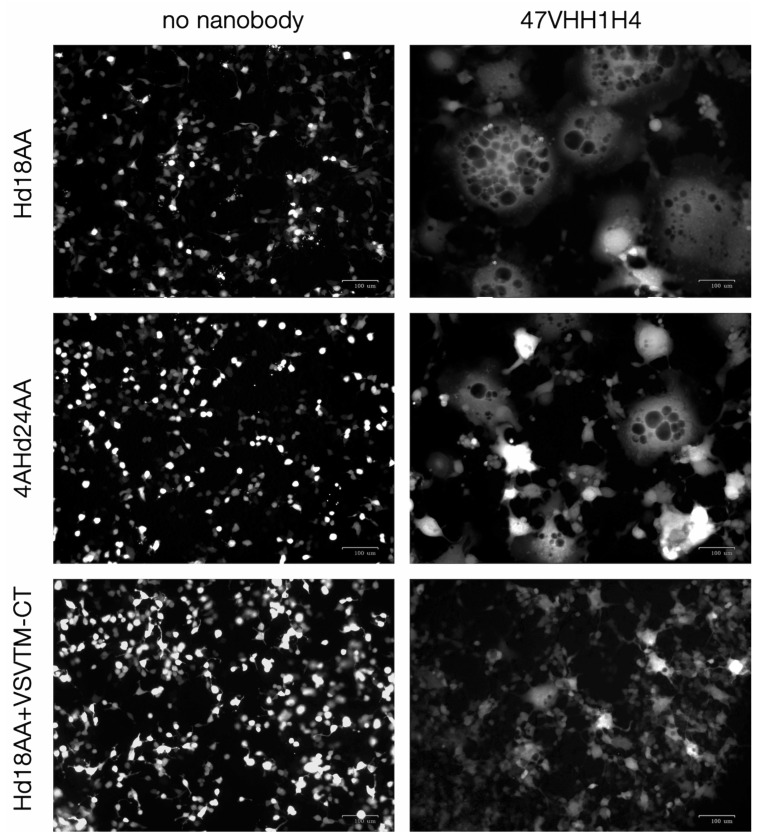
Syncytia formation after transfection with plasmids coding 47VHH1H4-retargeted measles glycoproteins. Left column—control samples, transfected with pCG-Hd18AA, pCG-4AHd24AA-XB, and pCG-VSVTM-CT + pCG-Hd18AA-XB. Right column—samples transfected with pCG-Hd18AA-47VHH1H4, pCG-4AHd24AA-47VHH1H4 and pCG-47VHH1H4-VSVTM-CT + pCG-Hd18AA-XB.
